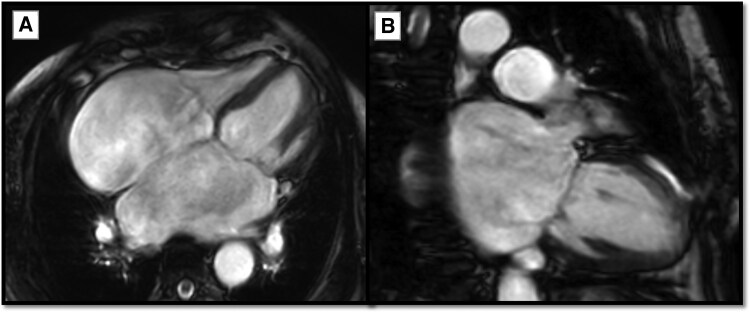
Cardiovascular magnetic resonance imaging steady state free precession (SSFP) images. (*A*) Four-chamber view SSFP image showing marked bi-atrial dilatation, small ventricular cavities, and restrictive configuration of the heart. (*B*) Two-chamber view SSFP image showing hugely dilated left atrium and small left ventricular cavity.
